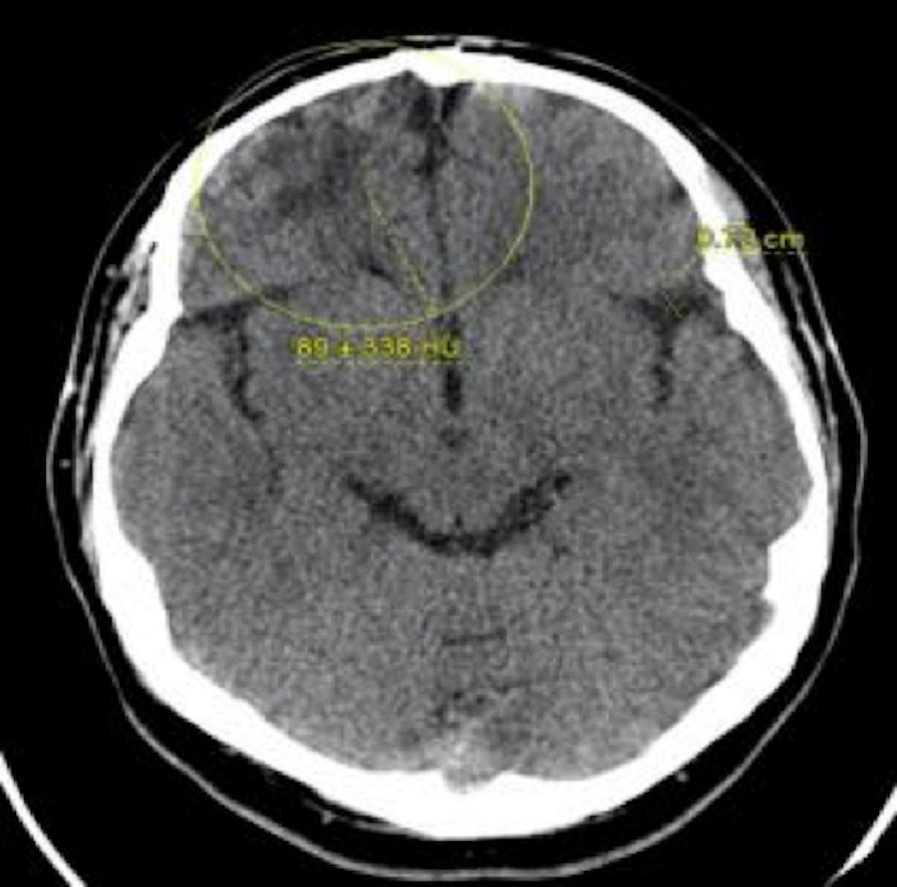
Computed tomography head without contrast coronal view. Yellow circle indicates area of encephalomalacia in right frontal lobe.
